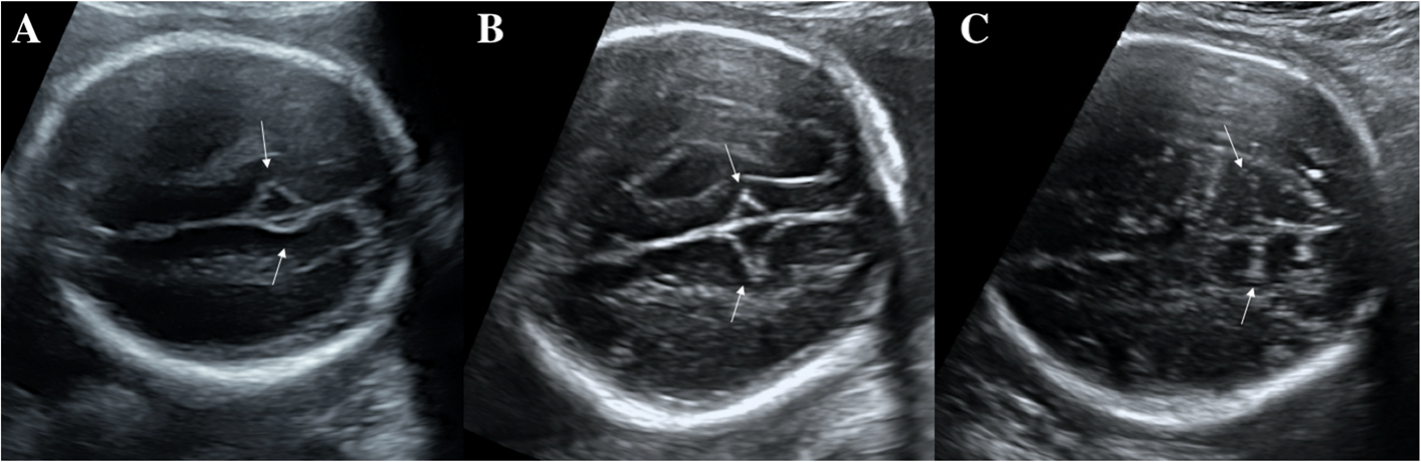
Asymmetry in the development and ‘catch-up growth’ of parieto-occipital sulcus in a IMVM fetus with unilateral ventriculomegaly. Ultrasound image of parieto-occipital sulcus on axial cephalic plane at 25 weeks (a), 28 weeks(b) and 31 weeks (c) of gestation. a The parieto-occipital sulcus was grade 1 on one side and was grade 2 on the other side. b The parieto-occipital sulcus was grade 2 on one side and was grade 3 on the other side. c The parieto-occipital sulcus was grade 4 on both sides
